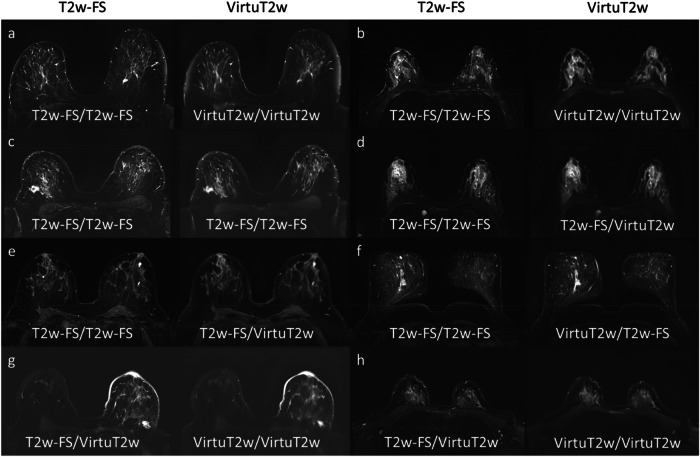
Example of image cases for which the two readers answered whether the respective image series looks like an original T2-weighted fat-saturated (T2w-FS) acquisition or a synthetic T2w-FS (VirtuT2w) image. The image names below each image indicate the respective readers’ answers, with Reader 1 presented on the left side and Reader 2 on the right side. In one case (c), both the readers mistook a VirtuT2w image for a T2w-FS. Notably, this image has a low level of blurring. In two cases (d, e), Reader 1 mistook the VirtuT2w image for a T2w-FS. In two cases, Reader 2 (g, h) mistook an original T2w-FS image for a VirtuT2w image. Both readers were able to identify the VirtuT2w images in most cases where significant blurring in the image was observed (a, b, f)
